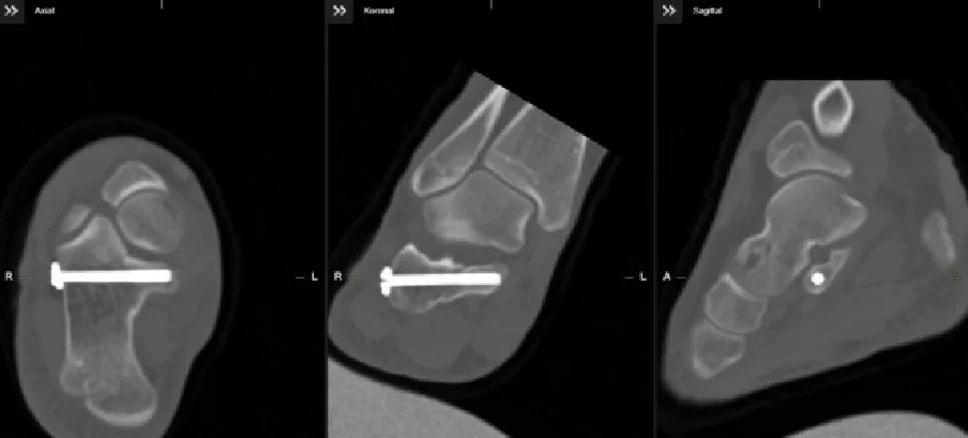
Intraoperative CBCT screw check for screw osteosynthesis of the sustentaculum tali
